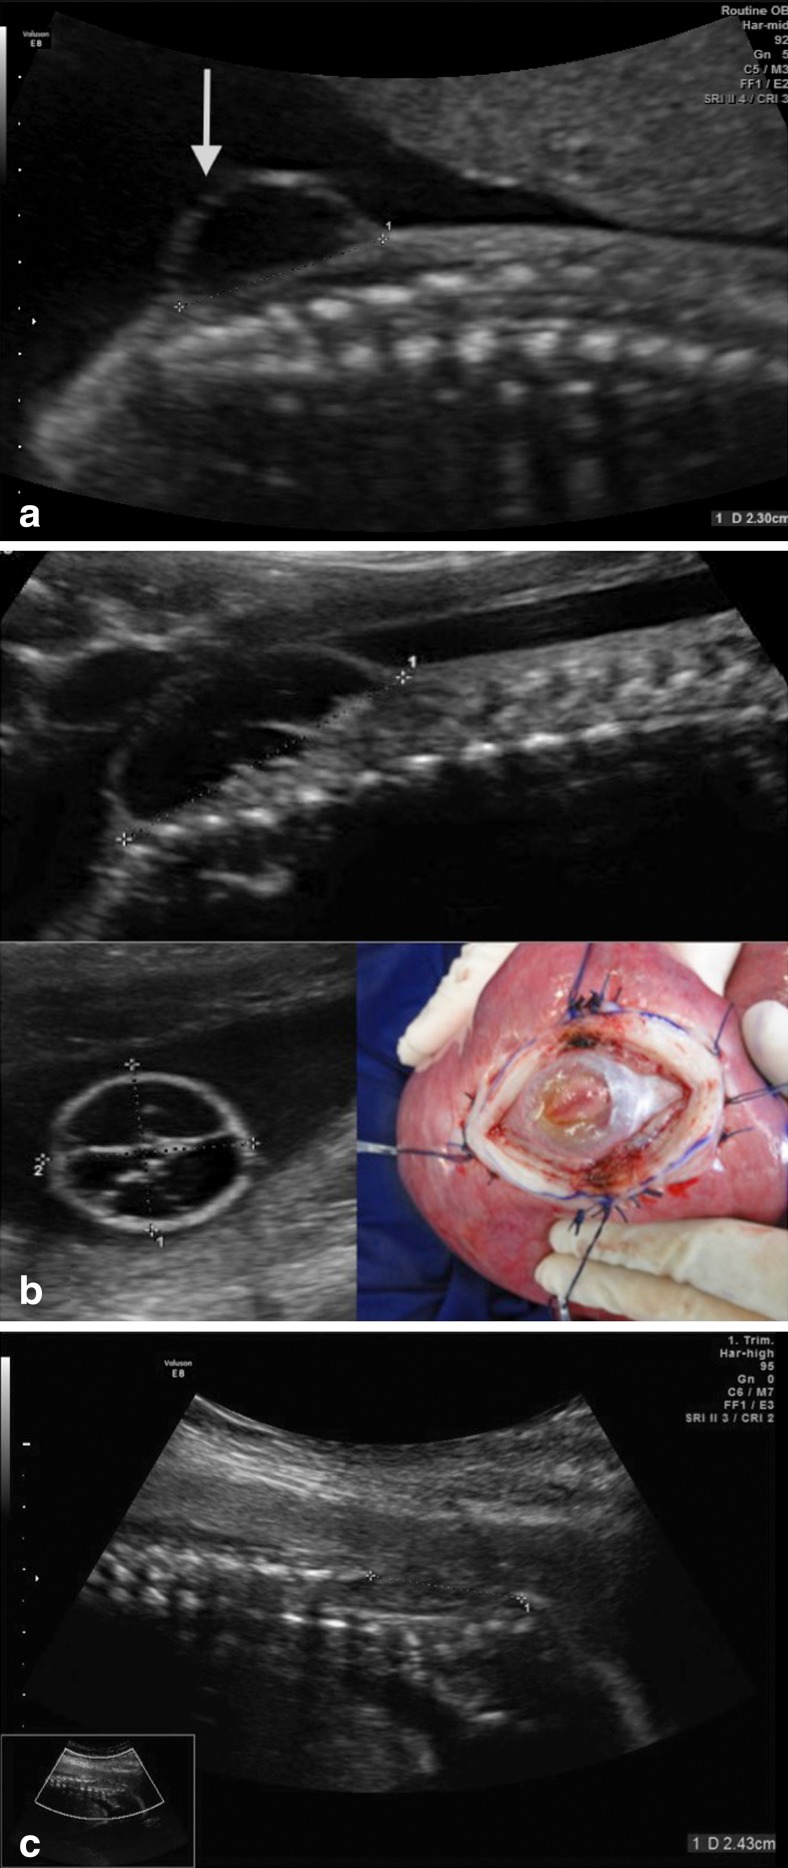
Two-dimensional ultrasound showing: a Meningocele. b Myelomeningocele with a neural tissue inside and the respective intraoperative view. c Rachischisis
